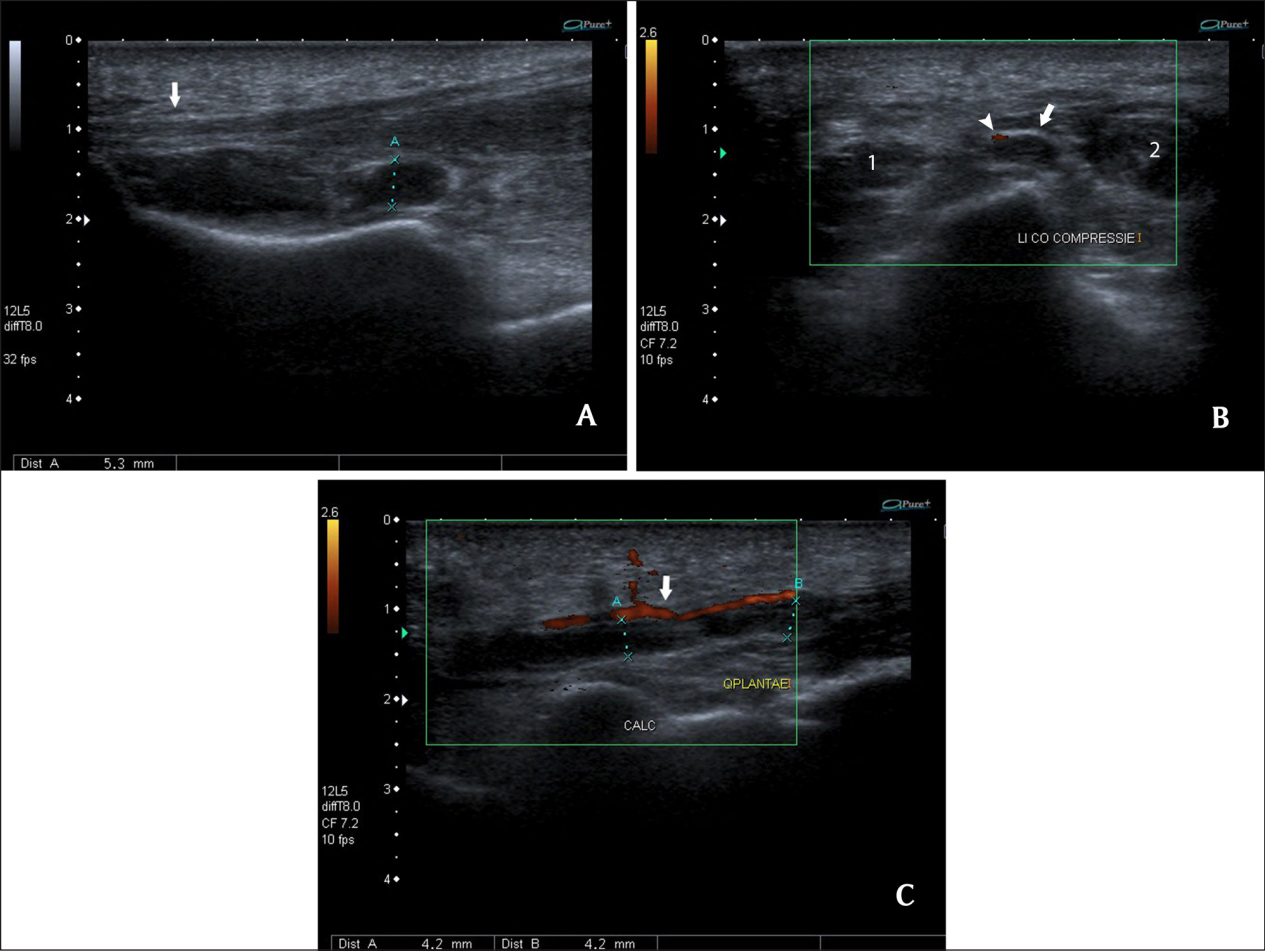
Ultrasound of the left foot. Sagittal view (A). There is a normal thickness of the plantar fascia between 2 and 4 mm (arrow). Tubular, hypoechoic structures are seen near the plantar fascia. Coronal view (B) with compression and color Doppler imaging. These structures are not compressible, and there is no blood flow. The thrombus (arrow) is located deeply and medially to the plantar artery (arrowhead) and between the musculus (m.) flexor digitorum brevis (1) and m. abductor hallucis (2). Sagittal view with color Doppler imaging (C). The thrombi (B-C) are located deeply beneath the medial plantar artery (arrow).
